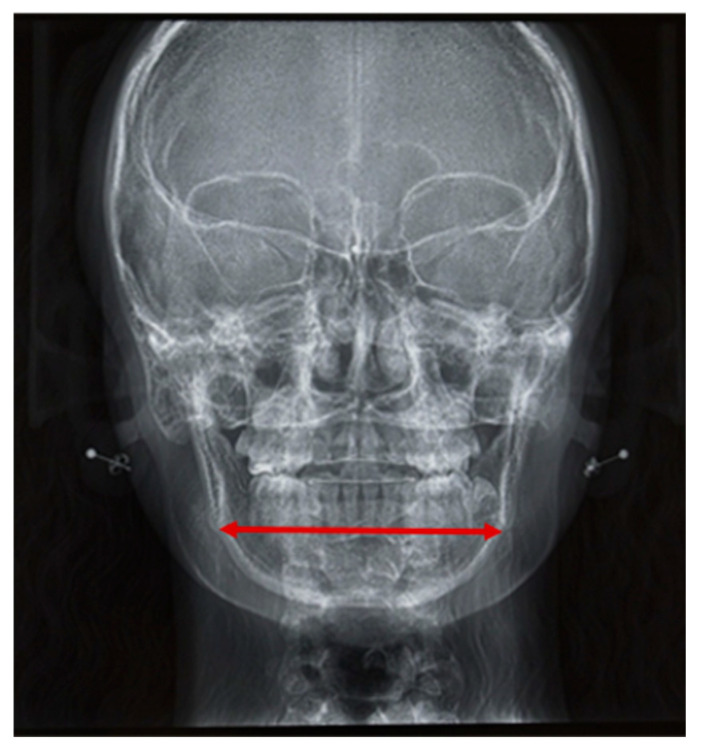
Determination of mandibular width on frontal radiographic cephalograms.
